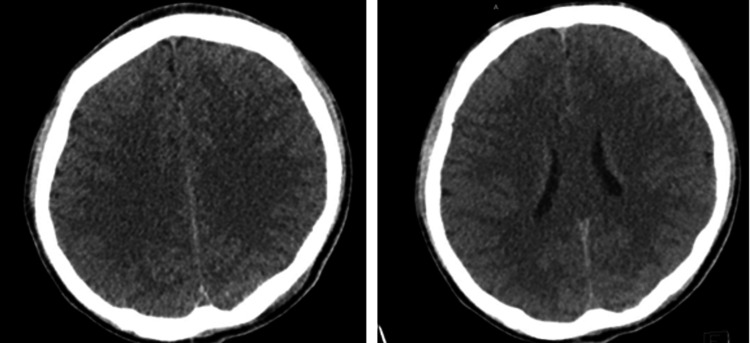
Computed tomography (CT) of the head. Initial CT of the head with representative slices showing no obvious abnormality.
